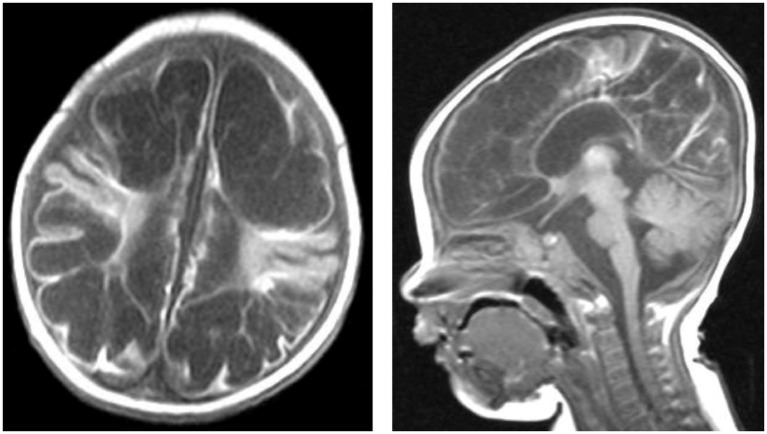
Follow-up cranial MRI plain scan: Thinning of the cerebral cortex in both hemispheres, widening and deepening of the sulci and cisterns, extensive encephalomalacia in both cerebral hemispheres, and dilatation of the supratentorial ventricles.
